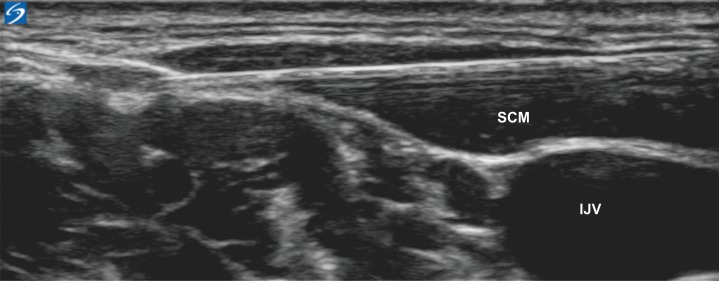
Ultrasound-guided superficial cervical plexus block. SCM: sternocleidomastoid muscle, IJV: internal jugular vein.
